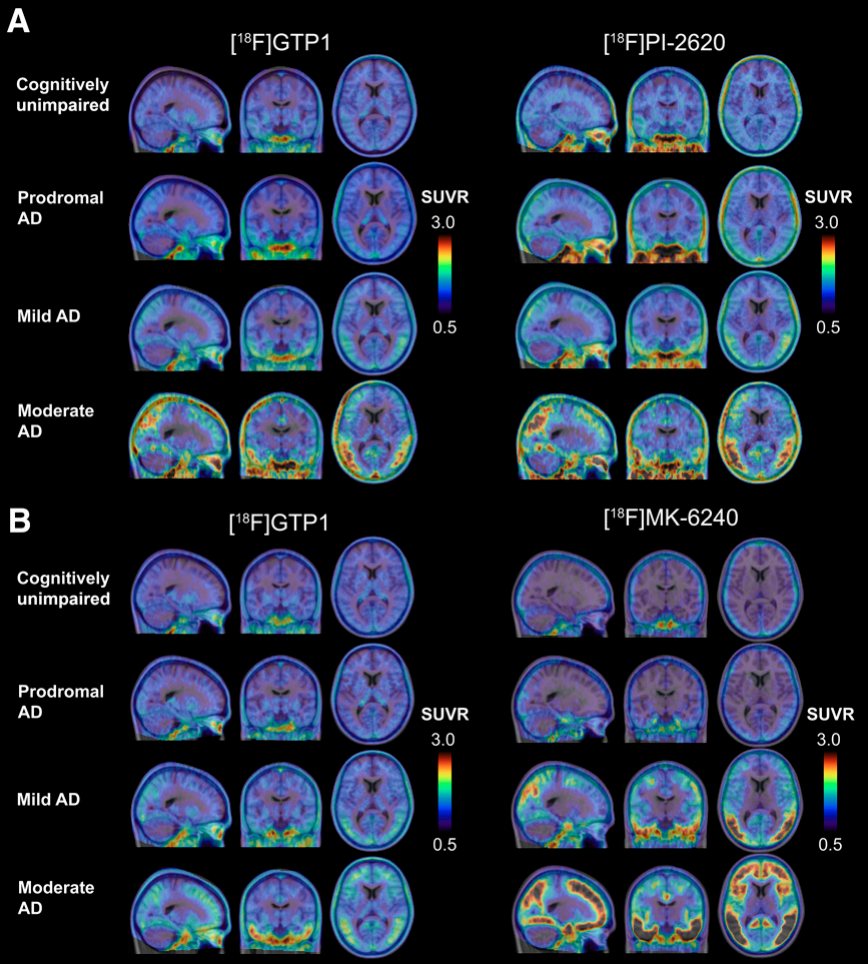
Average SUVR images from participants in cohort 1 (A), imaged with [^18^F]GTP1 and [^18^F]PI-2620, and cohort 2 (B), imaged with [^18^F]GTP1 and [^18^F]MK-6240, representing range of uptake in both cohorts. Each row contains average SUVR images from same participants.
